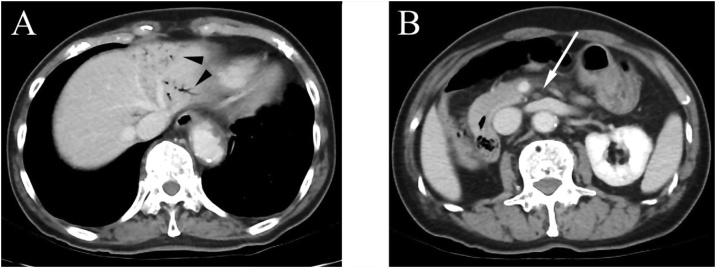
Initial enhanced computed tomography (CT) findings. A Black arrowhead: gas in hepatic portal veins. B White arrow: complete occlusion of the superior mesenteric artery.
